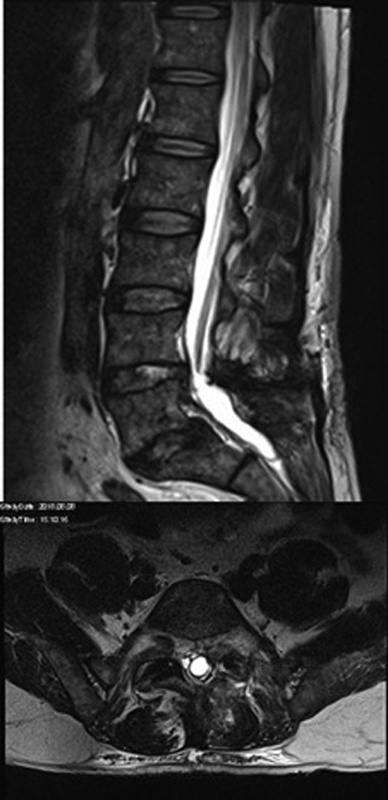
A T2-weighted magnetic resonance imaging (sagittal and axial view) performed after initial surgery showing a cystic enlargement of the dural sac from L5 to S2.
